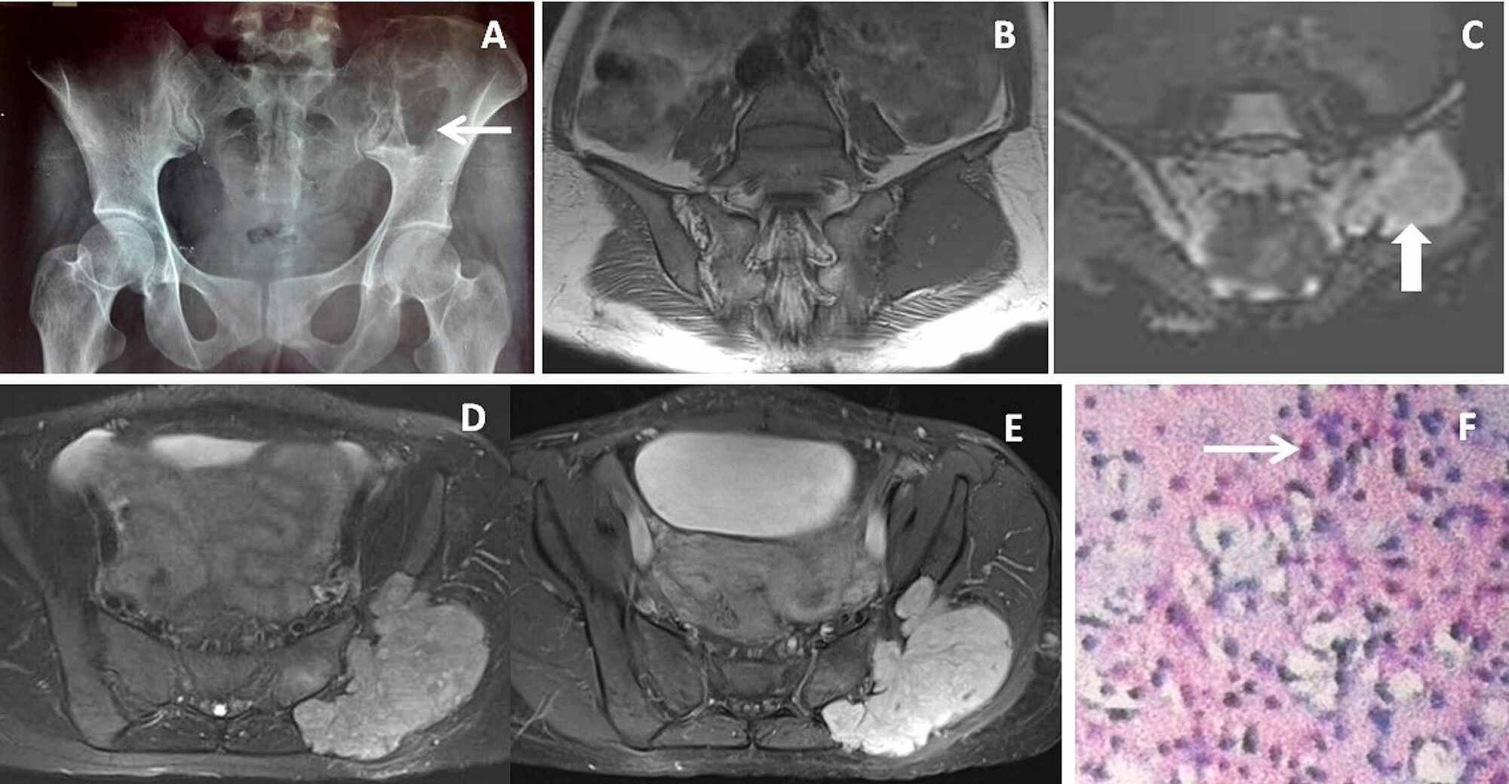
Iliac bone chondromyxoid fibroma Thirty-two-year-old female presented with pain and swelling in left buttock for two years. X-ray AP view of pelvis (A) showed ill-defined osteolytic lesion in left iliac bone (← arrow). Coronal T1 weighted (T1W) image  B ) showed a well-defined lobulated hypointense lesion in the left iliac blade. Coronal diffusion-weighted image (DWI) (C) shows diffusion restriction within the mass (↑ arrow). Axial proton density fat-suppressed (PDFS) image (D) showed the lobulated hyperintense lesion in the left iliac blade displacing adjacent gluteal muscles. Post gadolinium axial T1W image (E ) showed moderate homogenous enhancement of the lesion. 10X HPE image (F) showed lobules of spindle-shaped cells in abundant myxoid to chondroid stroma ( →arrow) suggesting chondromyxoid fibroma.
